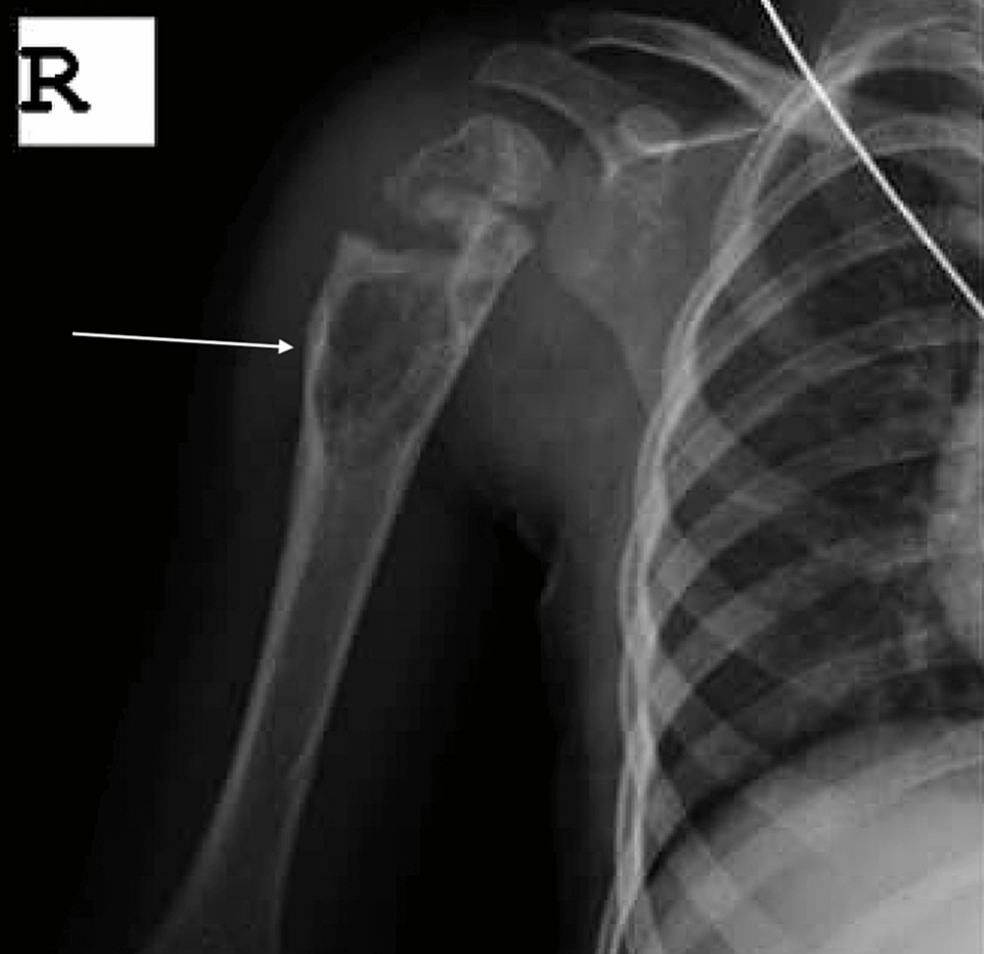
X-ray of the right proximal humerus
